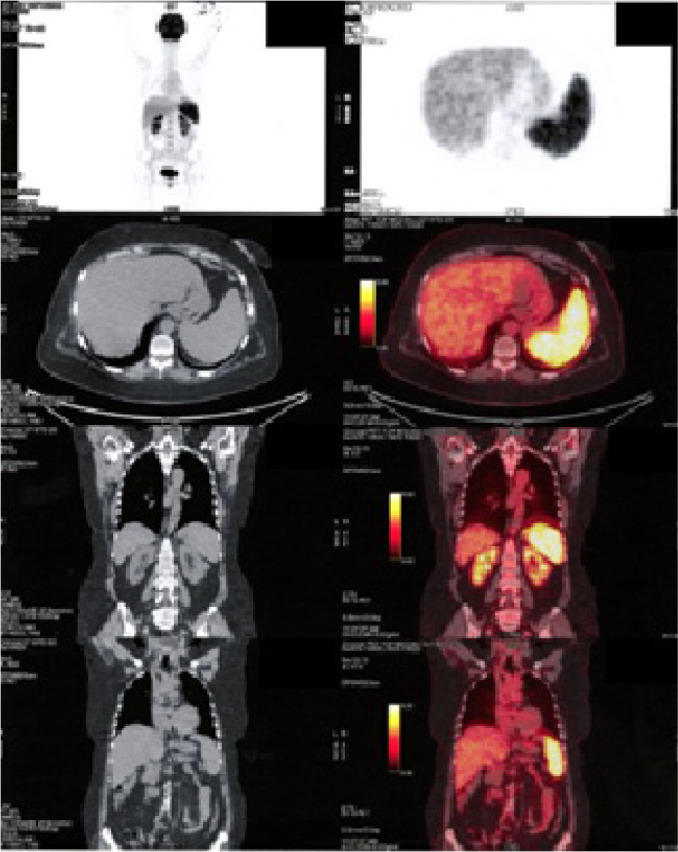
Positron emission tomography/computed tomography scan with fluorodeoxyglucose F18, showing increased metabolic activity of the spleen.
